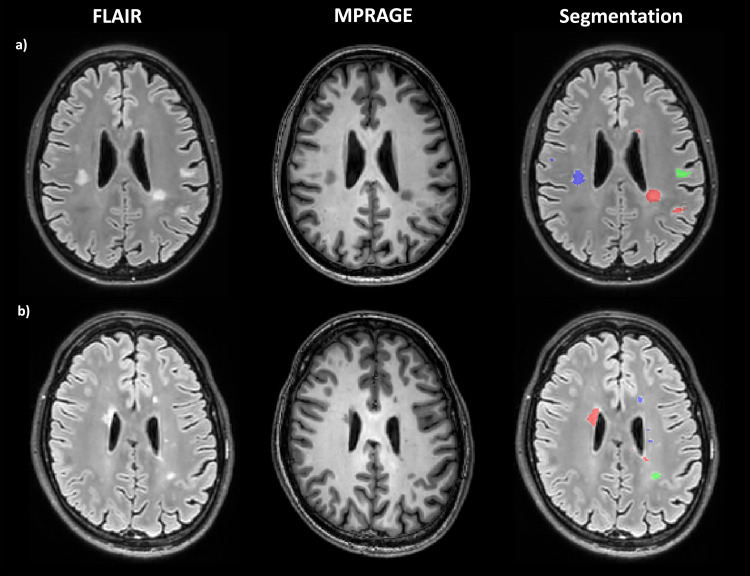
Representative lesion segmentation of two MS patients (a, b). FLAIR and MPRAGE images are provided for comparison. Orientation is indicated with R for right. Colors indicate location (red: periventricular; green: (juxta)cortical; blue: subcortical). FLAIR, fluid-attenuated inversion recovery; MPRAGE, T1w magnetization prepared rapid gradient echo.
